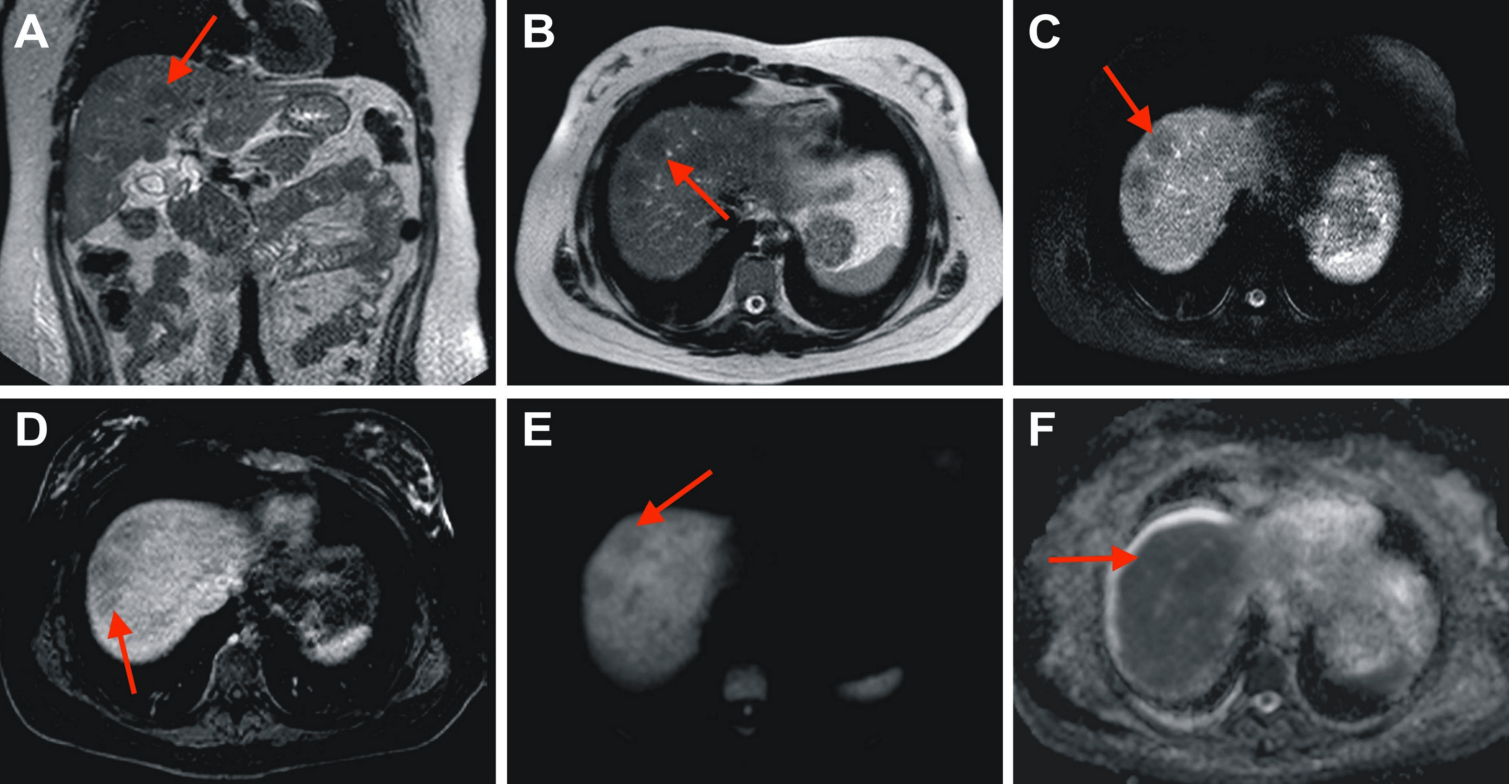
Abdominal MRI obtained during the patient’s initial evaluation Abdominal MRI with gadolinium contrast. (A) Coronal T2. (B) Axial T2. (C) Axial SPAIR. (D) Axial T1-weighted image with gadolinium (portal phase). (E) Axial diffusion-weighted imaging. (F) Axial apparent diffusion coefficient map. The liver is of preserved size and has regular contours, but it exhibits heterogeneous signal intensity due to multiple focal, nodular lesions (arrows). These lesions appear hypointense on T2/SPAIR sequences (A, B, C), isointense on T1, with no enhancement following intravenous gadolinium administration (D), and show no diffusion restriction (E, F).
